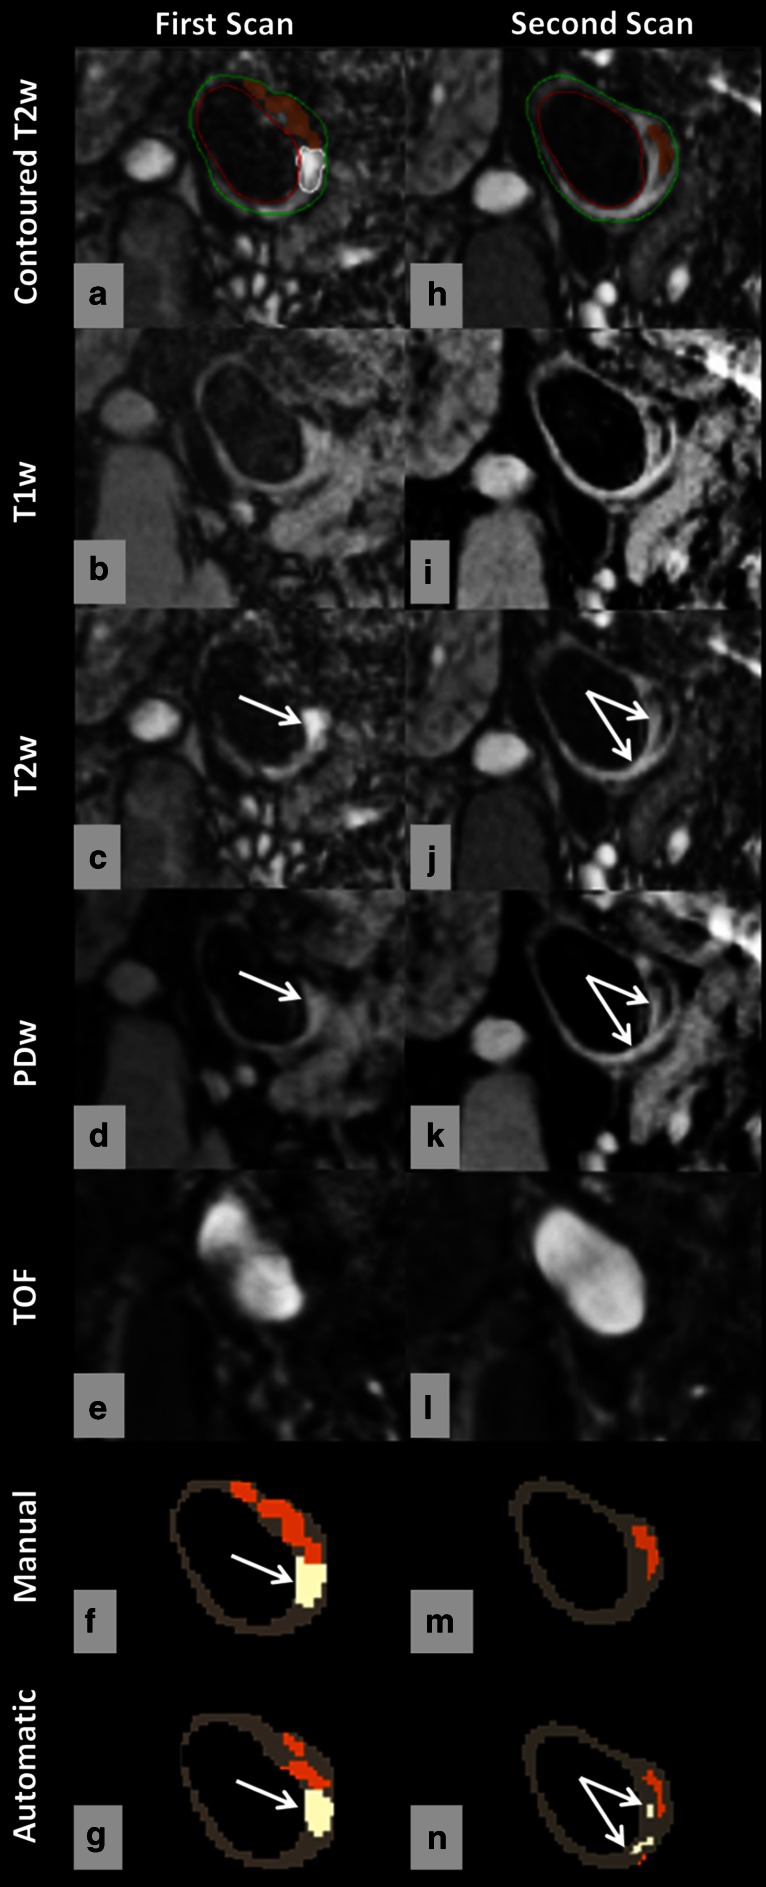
Manual and automated classification of loose matrix and calcification using multicontrast MR images, obtained in repeated scans. Manual contours overlaid on the T2w image (a, h). Loose matrix = *white*, calcification = *orange*, lumen = *red*, outer wall = *green*. Compared with the intensity of SCM muscle, regions with a hypointense signal on all four weightings are considered calcified tissue; while loose matrix (*white arrow*) is hyperintense on T2w and PDw image. As can be seen, calcification (*orange region*) could be detected with good repeatability in repeated scans by both segmentation methods (f, m, g, n); while loose matrix (*white regions*) could be detected with better agreement of size and location in two sessions by using automatic classification (g, n)
